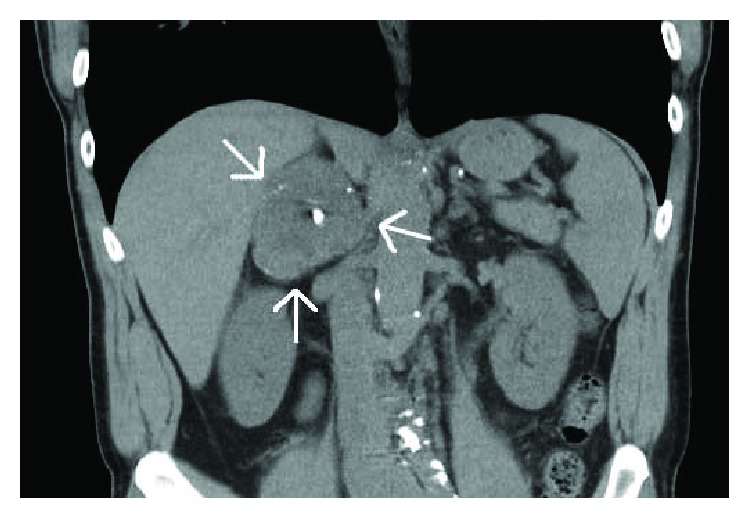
Adrenal-protocol CT abdomen, coronal demonstrates a 5 × 6.7 × 7 cm right adrenal mass (white arrows) with areas of calcification. Hounsfield units (HU): nonenhanced 36 HU (>10 HU indeterminate for adenoma), enhanced 84 HU, and delayed 73 HU. Absolute washout 22.9% (<60% indeterminate for adenoma) [3].
